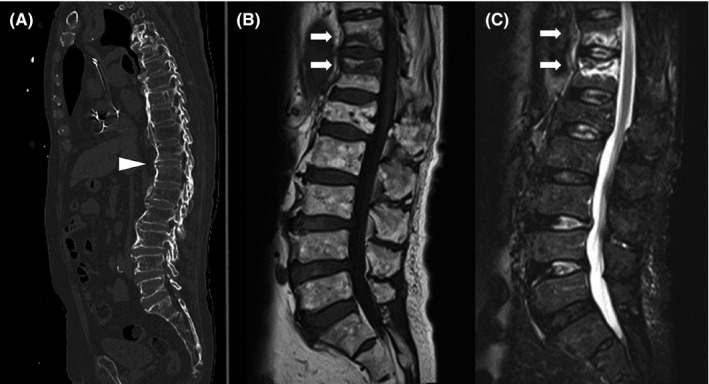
A, Fracture of the bone growth plate between the 11th and 12th thoracic vertebrae in a patient with DISH on a CT scan (arrowhead). B, Low‐intensity area in the 11th and 12th thoracic vertebrae on T1‐weighted images (arrow). C, High‐intensity area on the 11th and 12th thoracic vertebrae on T2‐weighted images (arrow)
